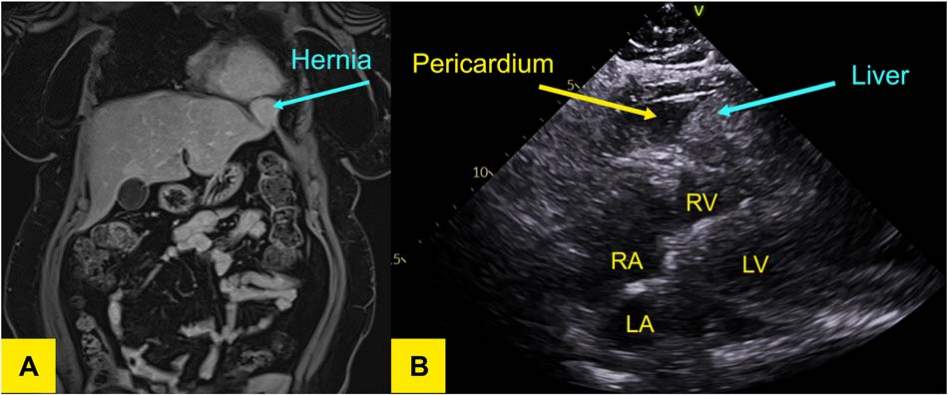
(A) T1-weighted MR of the liver in coronal plane showing a wide neck hernia at the anterolateral aspect of left hemidiaphragm that contains liver tissue and bulges outside the liver contour. No signal/enhancement abnormality. (B) Transthoracic echocardiogram demonstrating liver in pericardium adjacent to left ventricle.
